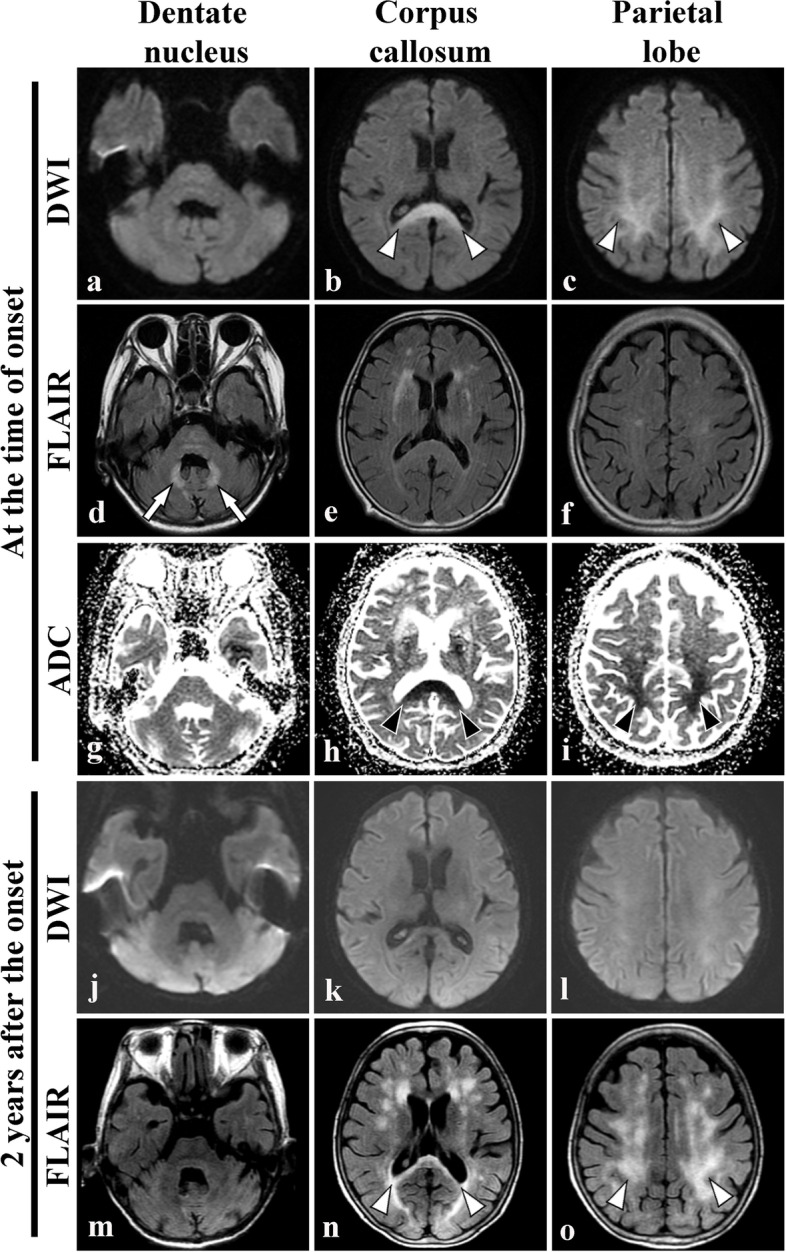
Brain magnetic resonance imaging (MRI) of the present patient with irreversible metronidazole-induced encephalopathy (MIE). a-c Diffusion-weighted imaging (DWI) at the time of onset shows hyperintensities in the splenium of the corpus callosum (white arrowheads, b) and the deep white matter of the bilateral parietal lobes (white arrowheads, c). d-f Fluid-attenuated inversion recovery (FLAIR) imaging of the brain shows hyperintensities in the dentate nuclei (white arrows, d), despite a lack of signal abnormalities in the corpus callosum and parietal deep white matter (e, f). g-i Apparent diffusion coefficient (ADC) values are reduced in the parietal lobes and splenium of the corpus callosum (arrowheads). Follow-up MRI of the brain 2 years after metronidazole withdrawal reveals near-complete resolution of abnormal intensities on DWI (j-l). m The abnormal signal intensities in the dentate nuclei have also disappeared. (n, o) However, widespread hyperintensities with diffuse brain atrophy are evident in the splenium of the corpus callosum and deep white matter including the parietal lobes on FLAIR (white arrowheads)
